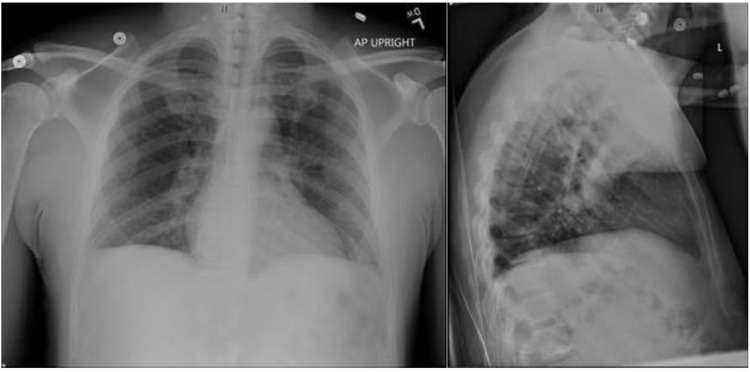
Chest X-Ray of Patient With Lymph Node Biopsy Demonstrating Sarcoidosis
